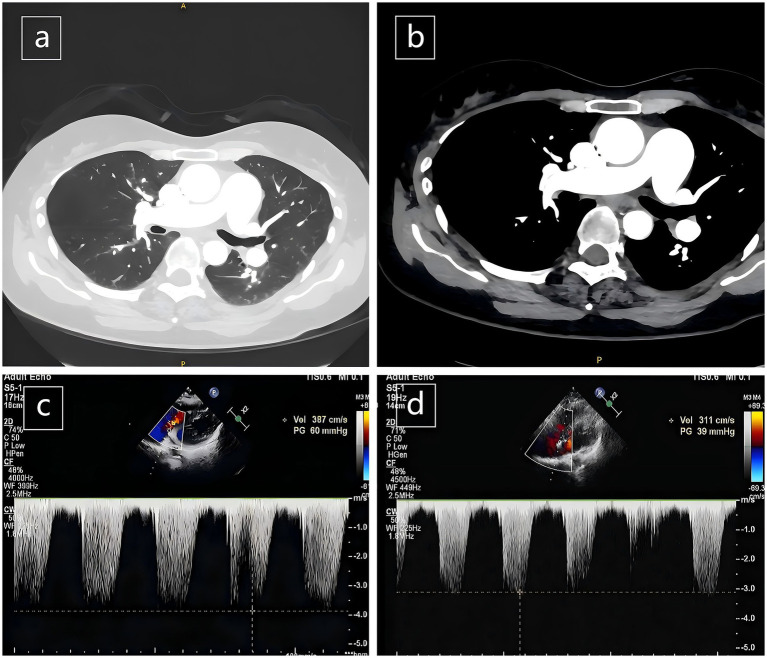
(a) The chest CT was largely unremarkable; (b) the CTPA did not detect any thrombi in the pulmonary arteries; (c) transthoracic echocardiography before NIPPV revealed severe pulmonary hypertension with an estimated PASP of 60 mmHg; (d) follow-up after NIPPV treatment demonstrated substantial regression of PASP to 39 mmHg.
